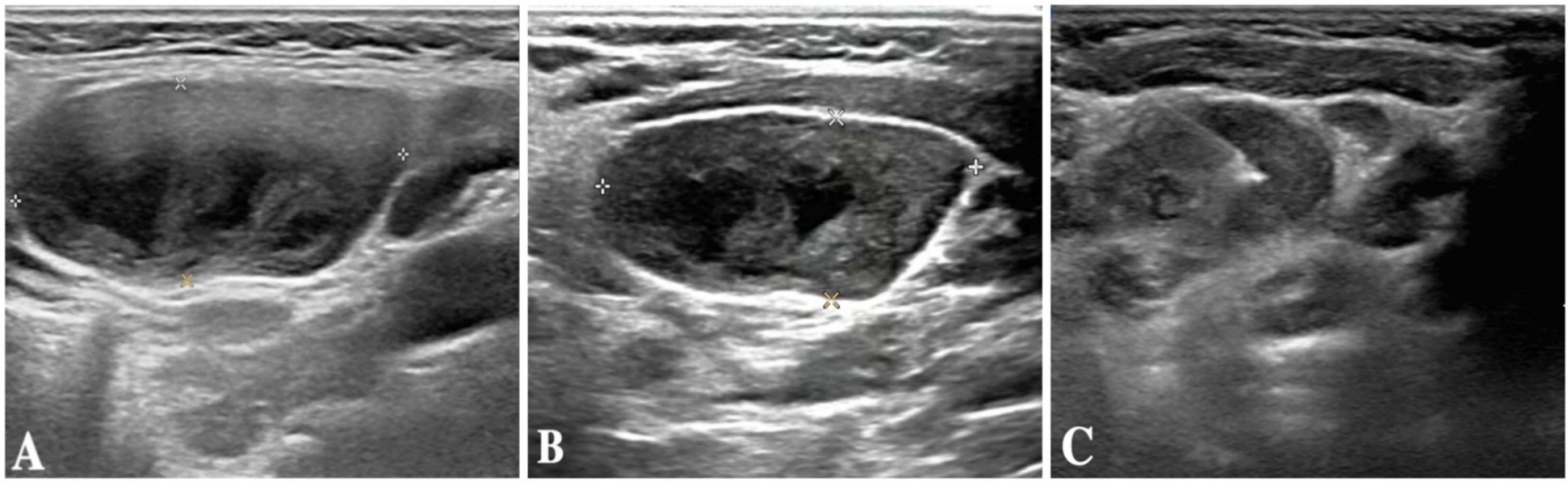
(A) Neck ultrasound results of the patient on August 7, 2024. (B) Neck ultrasound results of the patient on August 12, 2024. (C) Neck ultrasound results of the patient on August 15, 20.
